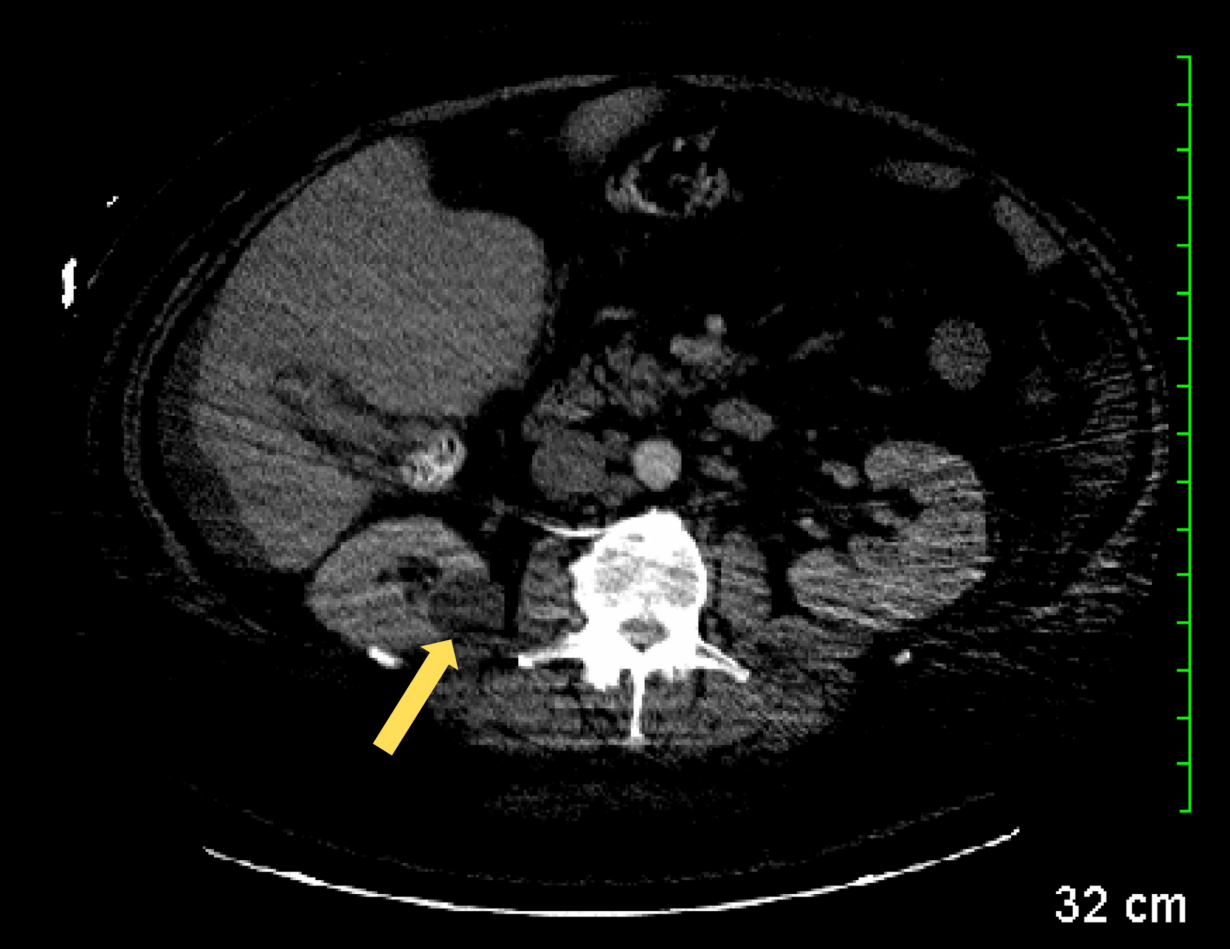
CT scan revealing a right renal cyst (yellow arrow). CT: computed tomography
